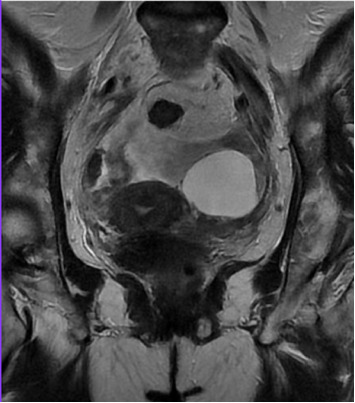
MRI T2 sequence, in a coronal view showing a complex tumor dependent on the left ovary.
